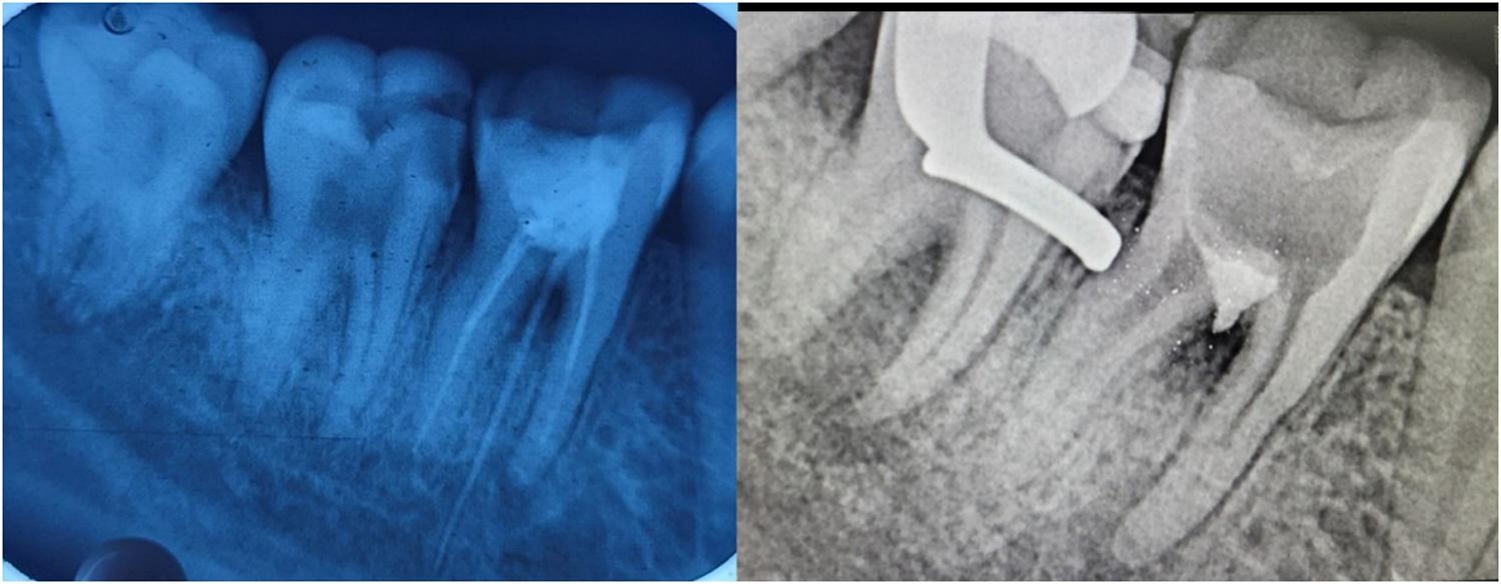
Preop & postop IOPA radiographic representation of perforation repair with the use of biodentine.
